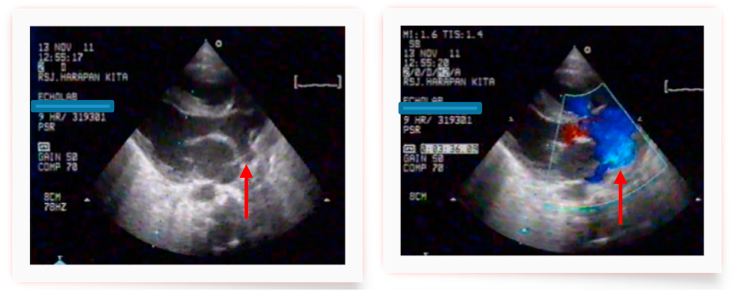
Long Axis view revealed a defect at AORPA *(Arrow)* from 2D Echocardiography and colour Doppler. (For interpretation of the references to colour in this figure legend, the reader is referred to the Web version of this article.)
